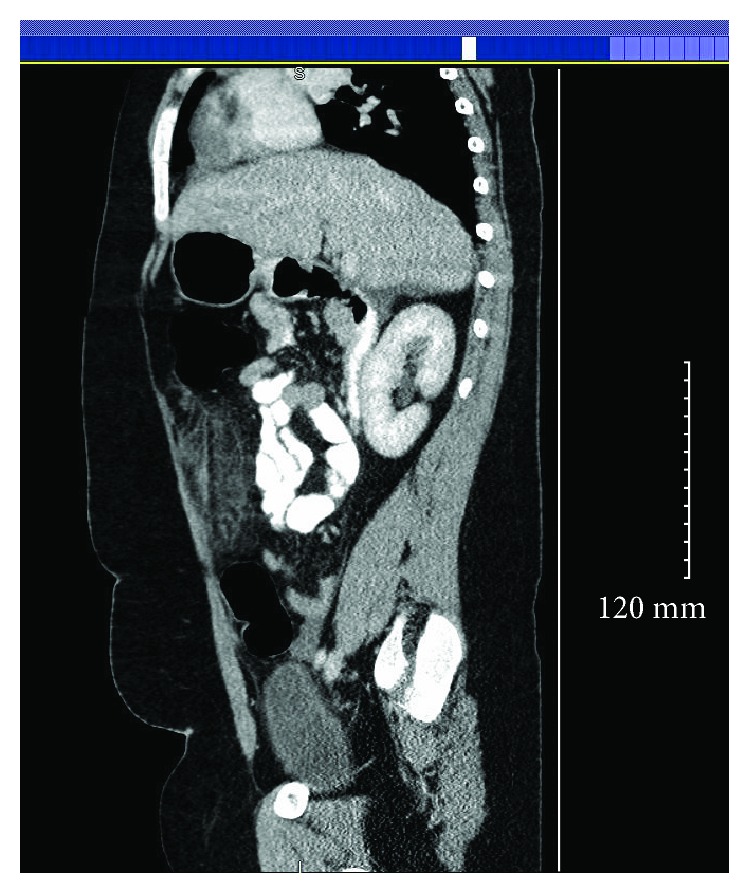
Sagittal CT indicating omental inflammation and fat stranding.
